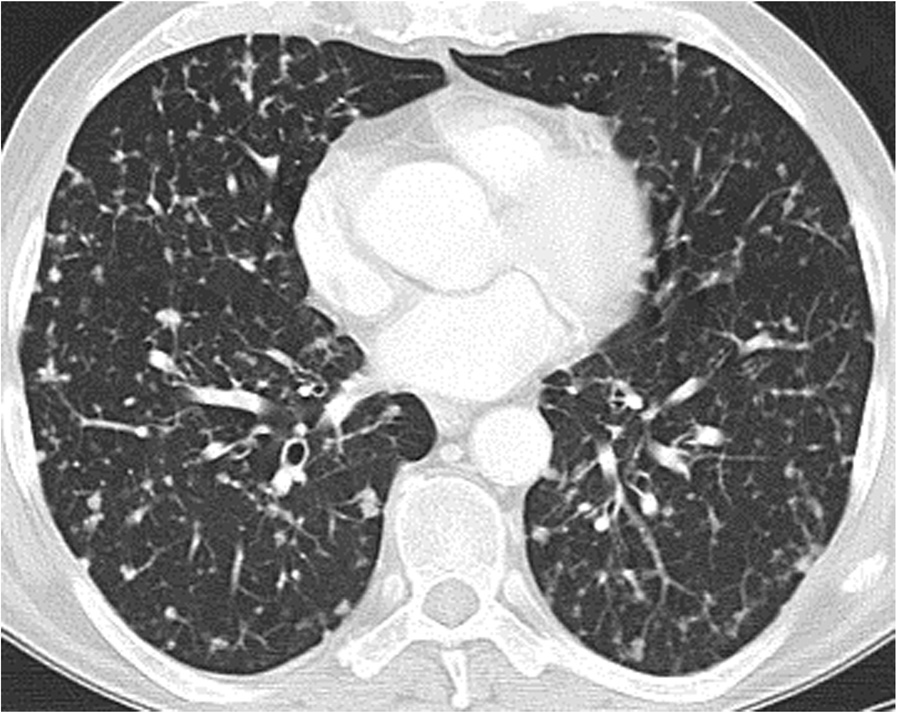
Multiple pulmonary metastases. Patient with sigmoid carcinoma. Chest CT at the level of the right inferior pulmonary vein at lung window: Multiple small pulmonary nodules with different sizes and random distribution in all lung lobes
